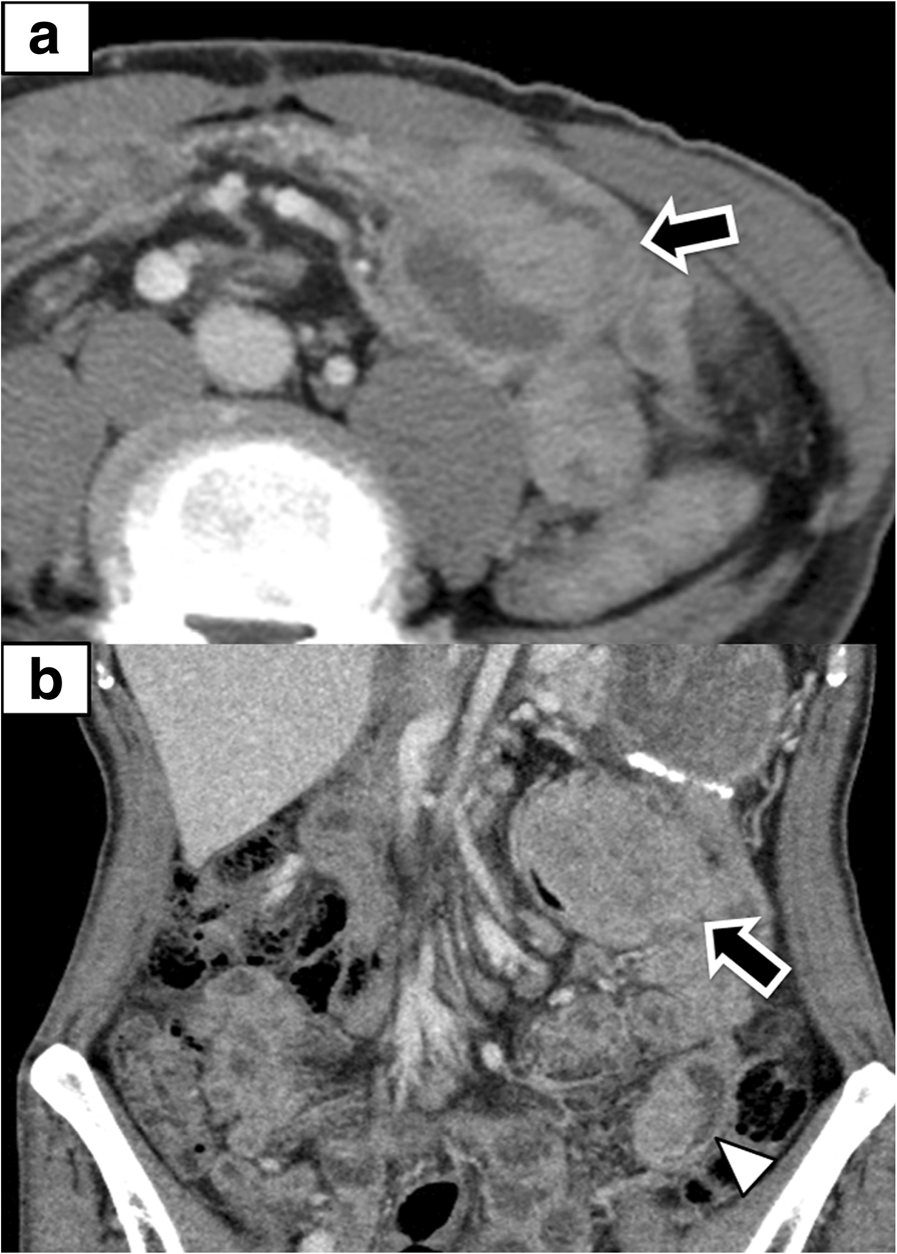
a Contrast-enhanced CT showing a mass, 55 mm in diameter, alongside the transverse colon. b Contrast-enhanced CT showing that the mass alongside the transverse colon enlarged to 65 mm, and an additional mass, 25 mm in diameter, was found in the jejunum. Black arrow—the lesion alongside the transverse colon; white triangle—the jejunal lesion
